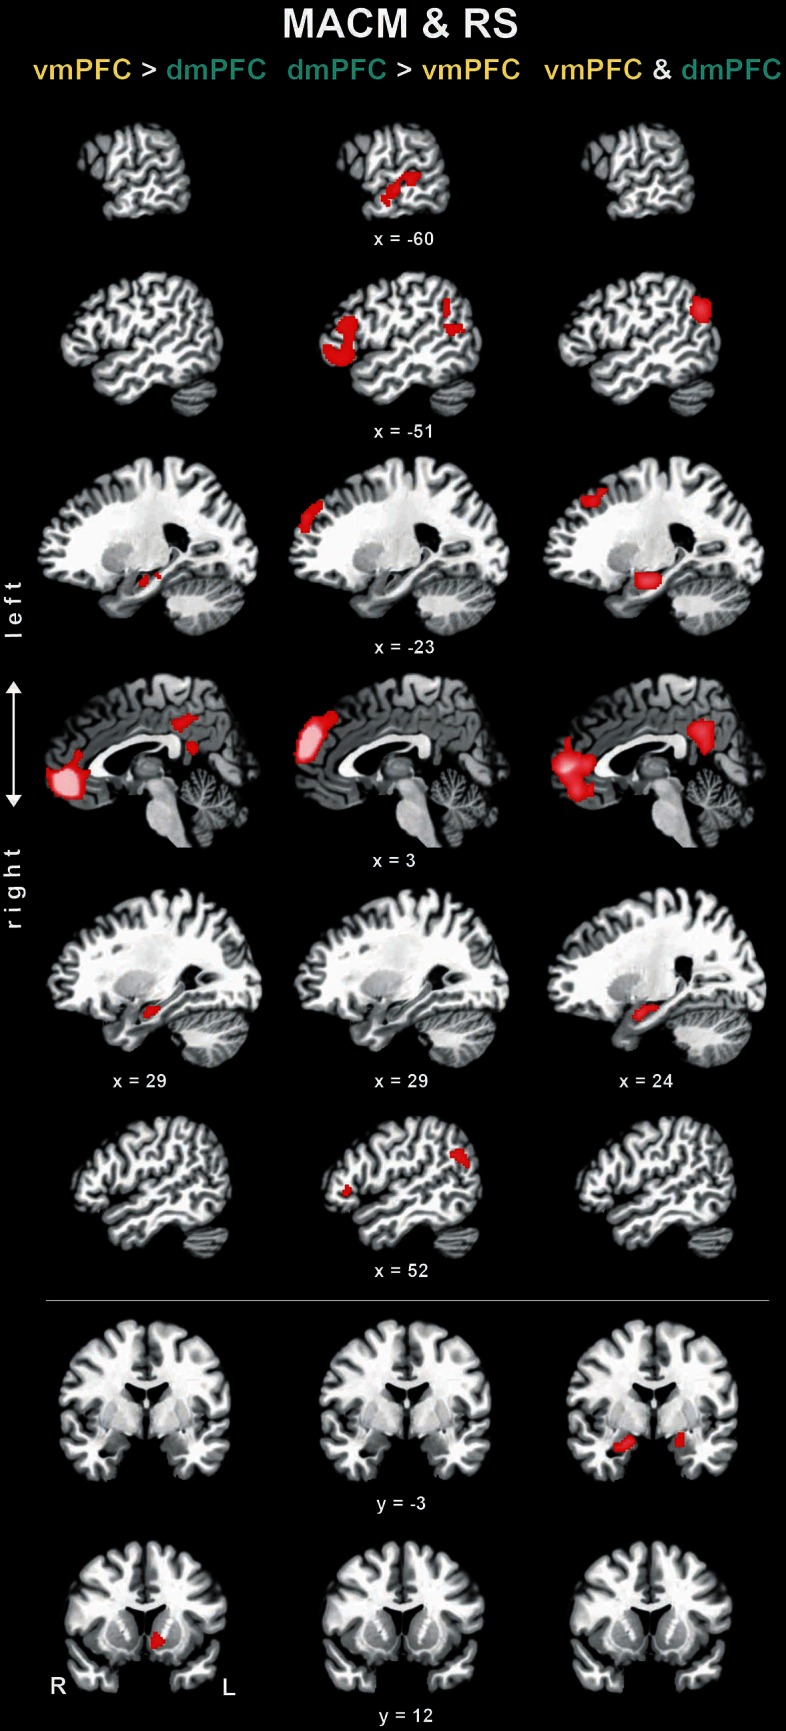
Difference and conjunction analyses based on congruent functional connectivity of the vmPFC and dmPFC seeds. Depicts sagittal and coronal brain slices of areas consistently more strongly coupled (left and middle column) with either seed or congruently coupled with both seeds (right column) across meta-analytic connectivity modeling (MACM) and resting-state (RS) analyses. Please refer to Table 3 for activation coordinates. All slices were created using mango (multi-image analysis GUI; http://ric.uthscsa.edu/mango/) on a T1-weighted MNI single subject template. Coordinates in MNI space. </>, difference analysis; &, conjunction analysis; R, right; L, left.
